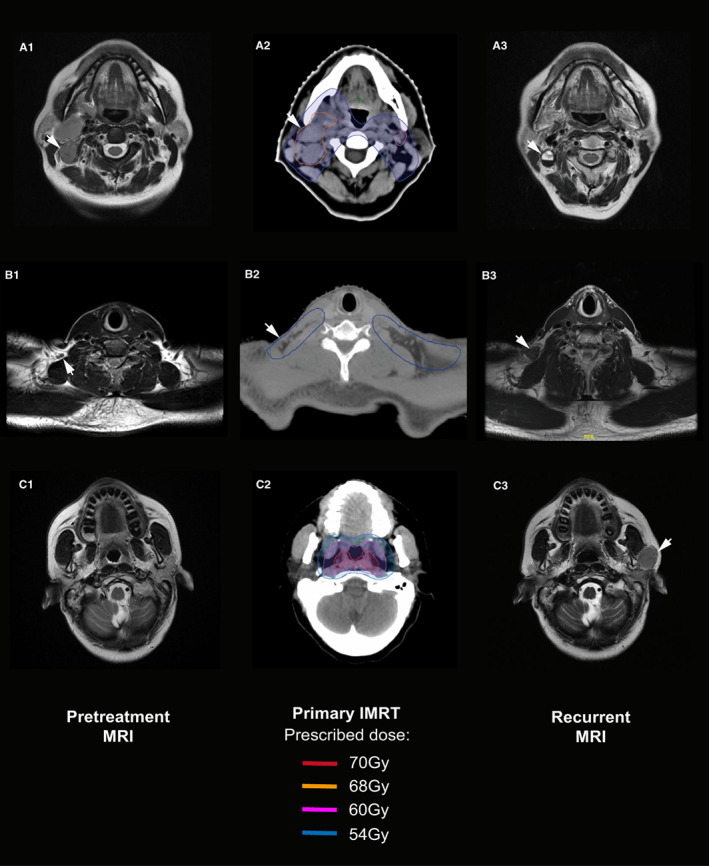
Three patients with solitary lymph node (LN) recurrence: in‐field failure at level IIb with a prescribed dose of 68 Gy (A1–A3); in‐field failure at level Va with a prescribed dose of 54 Gy (B1–B3), and out‐field failure in the parotid region (C1–C3). Left: Initial diagnostic magnetic resonance imaging (MRI). Middle: Target volume delineation and dose prescription of primary intensity‐modulated radiotherapy. Right: MRI at recurrence. Arrows indicate the recurrent LNs and pretreatment LNs re‐located at the same site on the diagnostic MRI.
